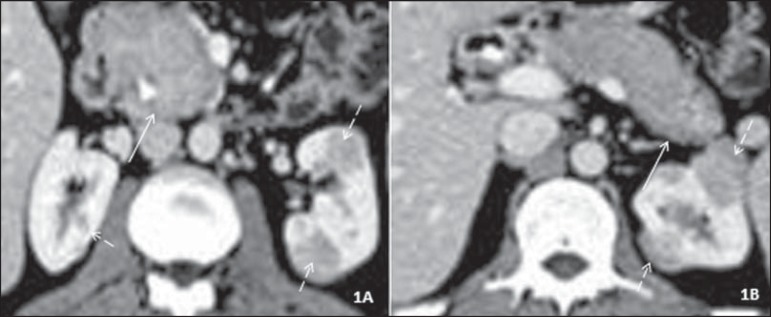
Axial contrast-enhanced CT scan of the abdomen, nephrographic phase.
